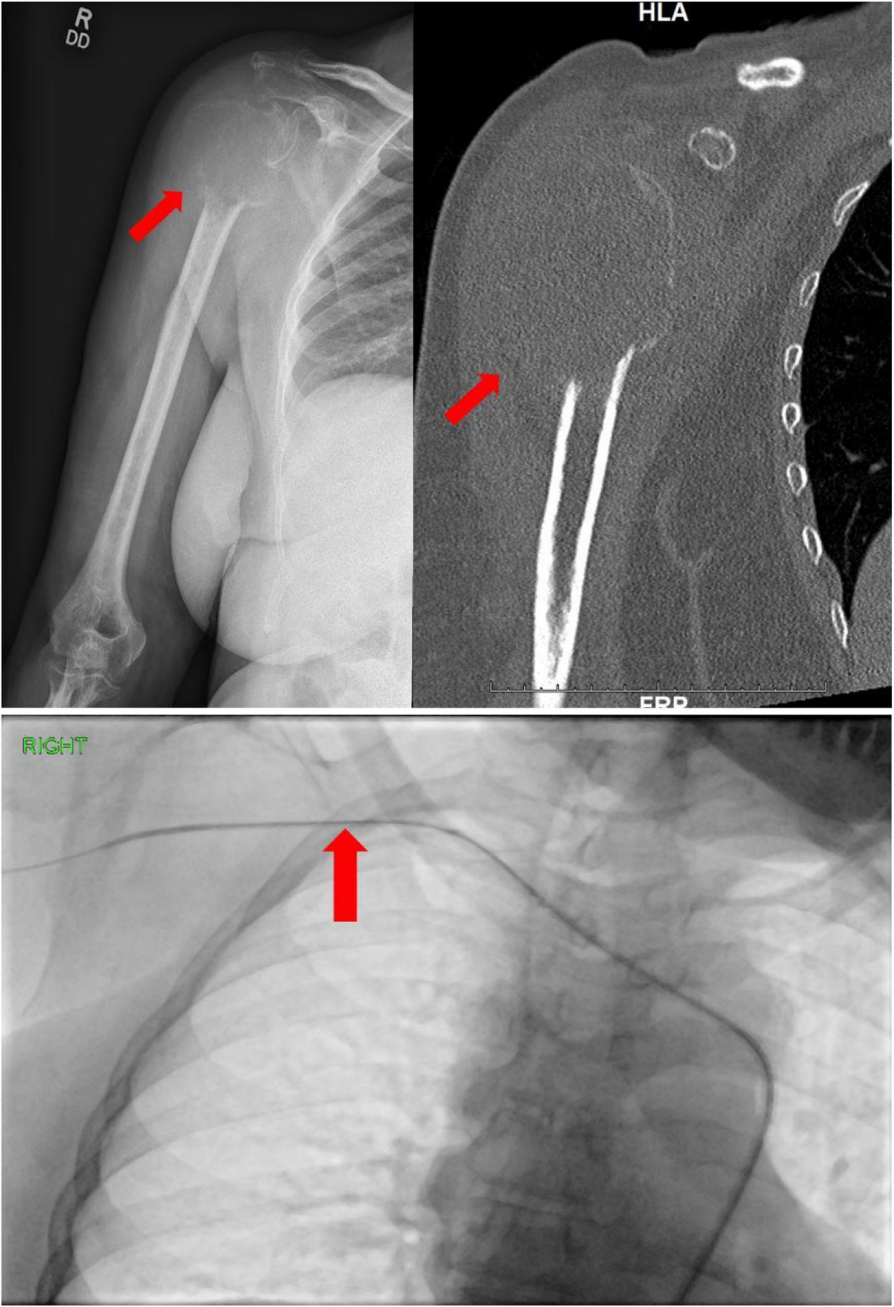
(A) Frontal x-ray and (B) coronal CT of the right shoulder show a large expansile lytic tumor in the humeral head, with an associated pathologic axial fracture of the surgical neck (arrow). (C) Intraprocedural spot fluoroscopic image demonstrates a 5F angled catheter in the right axillary artery from a femoral approach (arrow).
